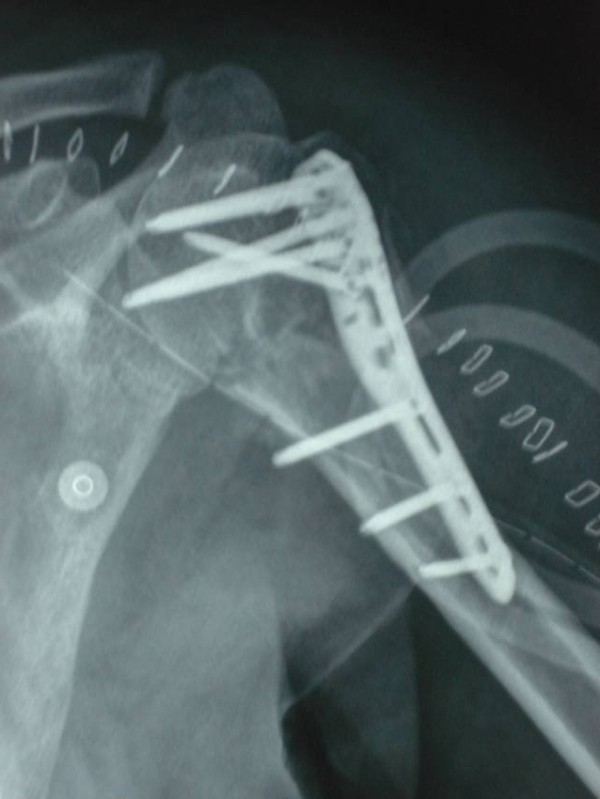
Immediate post operative X-ray in a patient showing primary screw perforation.
